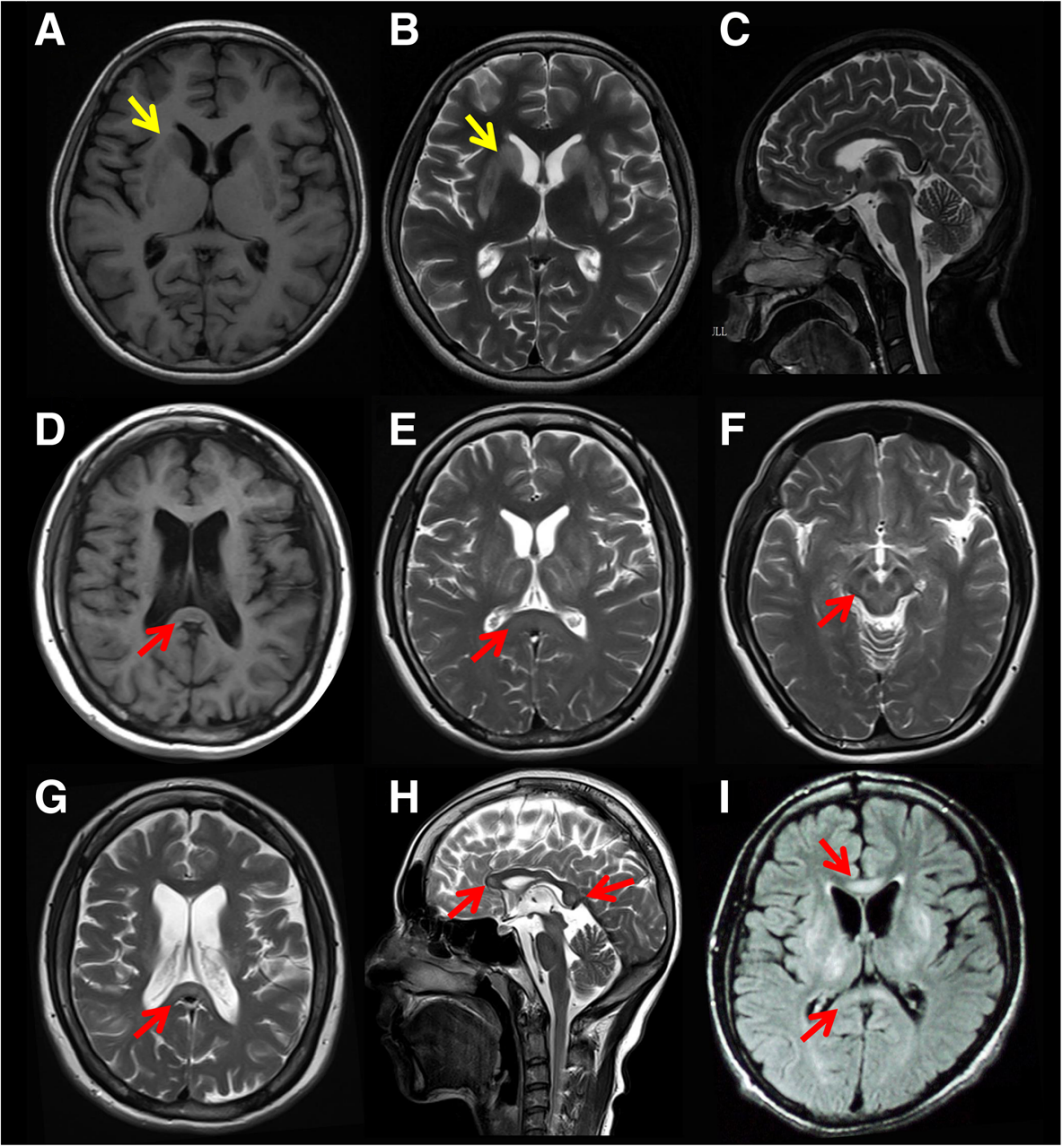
Representative MR image of WD with (WD-CCA) and without corpus callosum abnormalities (WD-no-CCA). a-c Representative MR image of WD without corpus callosum abnormalities. d-i Representative MR image of WD without corpus callosum abnormalities. a-c Bilateral, symmetrical abnormal signals of caudate nucleus and putamen in T1- and T2-weighted axial sequence MRI (yellow arrow). d T1-weighted axial MRI demonstrates that bilateral anterior horn of lateral ventricle enlarged and hypointensities in Splenium. e T2-weighted axial MRI demonstrates: bilateral, symmetrical hyperintensities in lenticular nucleus, thalamus and hyperintensity in Splenium. f T2-weighted axial MRI demonstrates face of the giant panda in brain stem, g T2-weighted axial MRI demonstrates hyperintensities in Splenium. h T2-weighted sagittal MRI demonstrates hyperintensities in the genu and splenium. i Fluid-attenuated inversion recovery sequence, axial MRI demonstrates hyperintensities in genu and splenium, and bilateral, symmetrical hyperintensities in putamen, globus pallidus, caudate nucleus and thalamus
